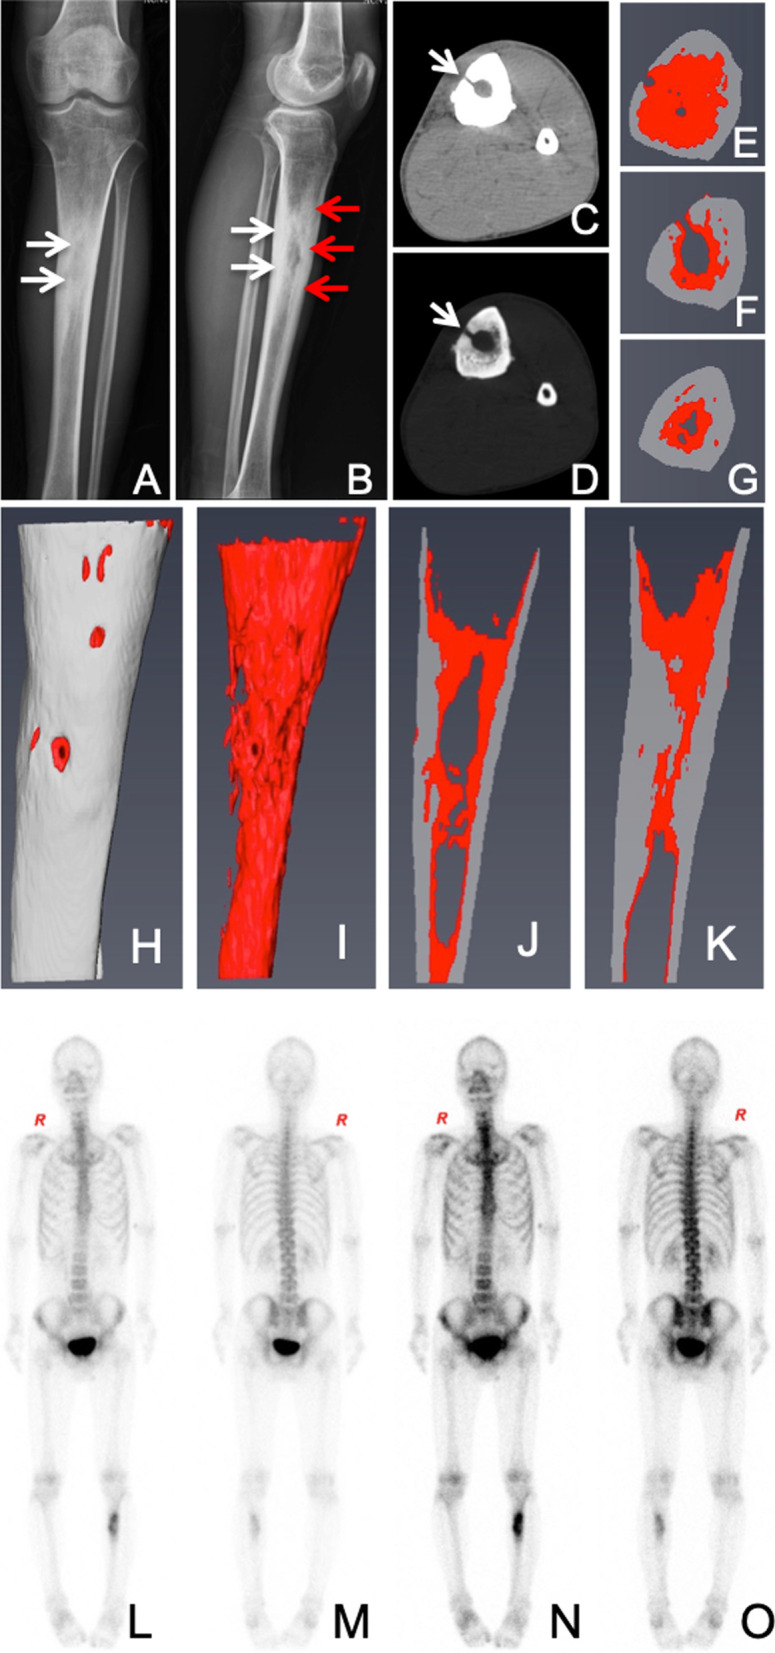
Preoperative imaging evaluation determined osteomyelitis lesion range and severity in 2012. The plain radiography of left tibial approximal diaphysis showed a central area of radiolucency with a surrounding thick rim of reactive bone sclerosis (A, B white arrows). CT scan found a cloaca (C, D white arrows) and adjacent reactive bone (E–G, red areas) from lesion area of Panel B (red arrows). Then we performed 3D reconstruction analysis by using a software Amria to determine the reactive bone within the intramedullary (H tibial; I intramedullary reactive bone; J, K sagittal section of tibia). The infection was confirmed by the emission computed tomography (ECT) (L–O)
